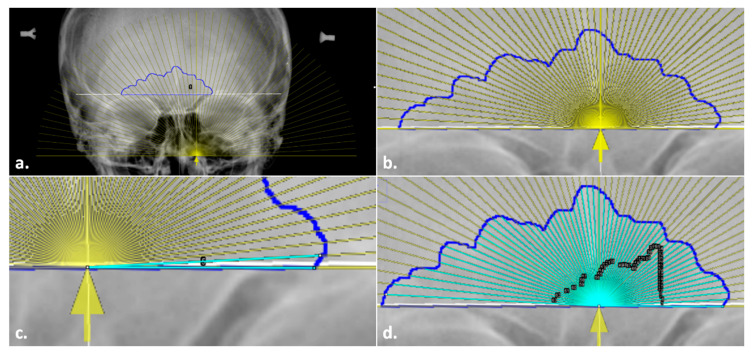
Placing the overlay in the modified steps in the Total Difference Method: (a) insertion of a semi-transparent overlay (yellow) onto the radiograph with the sinus outline and origin marked; (b) alignment of the overlay baseline and origin arrow (yellow) with the sinus outline (blue) and midline (underlying white solid line); (c) preliminary angle drawn at 3°; (d) completed angles with black ROI Manager numbered labels for editing, if required.
